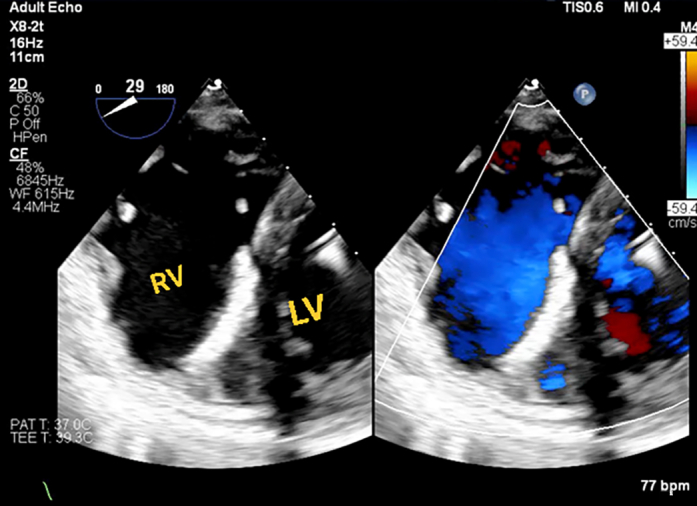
Final Transesophageal Echocardiogram Transesophageal echocardiogram at the end of the procedure showing no significant shunt. Abbreviations as in Figure 2.
